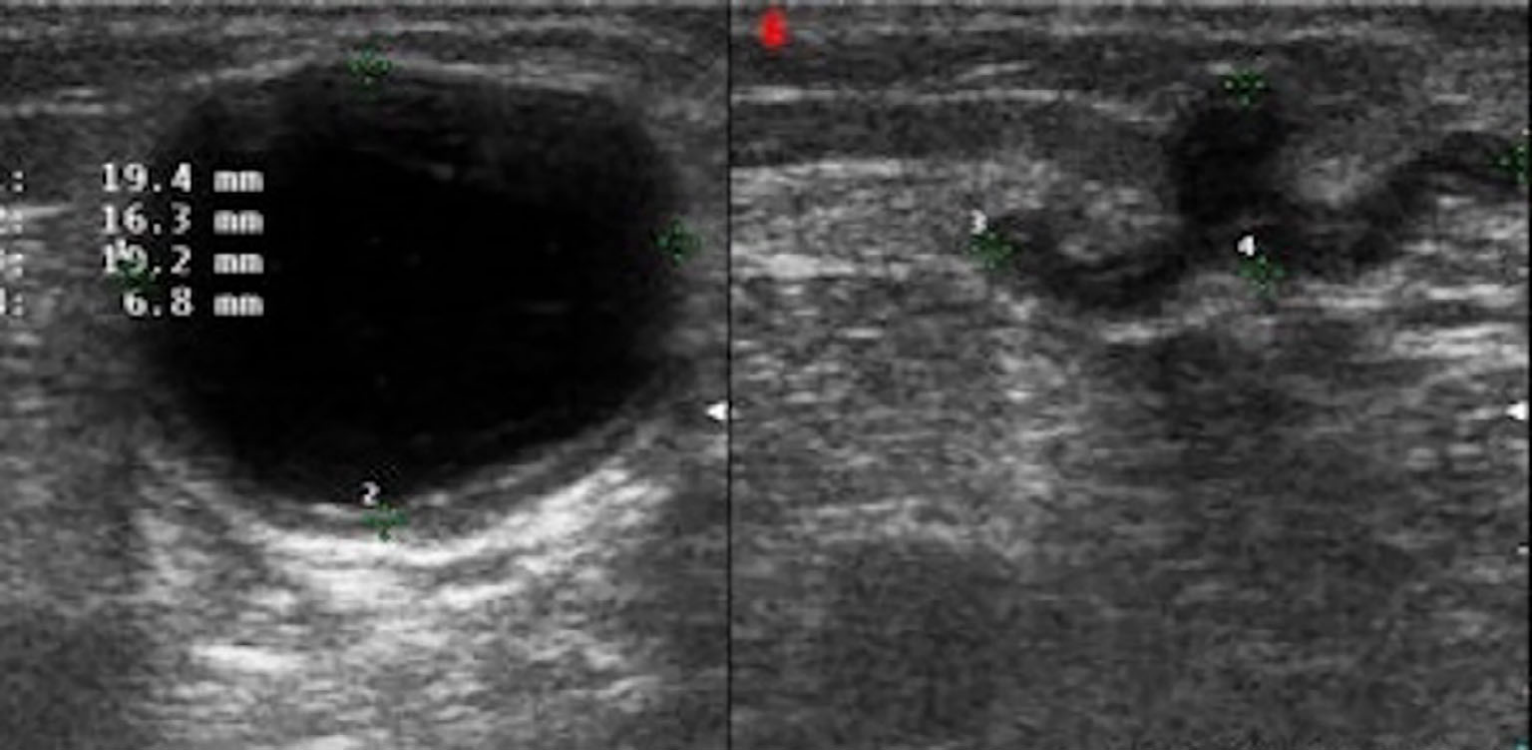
Ultrasound (US)‐guided seroma aspiration. US scan before and after percutaneous aspiration of the 2‐cm collection.
